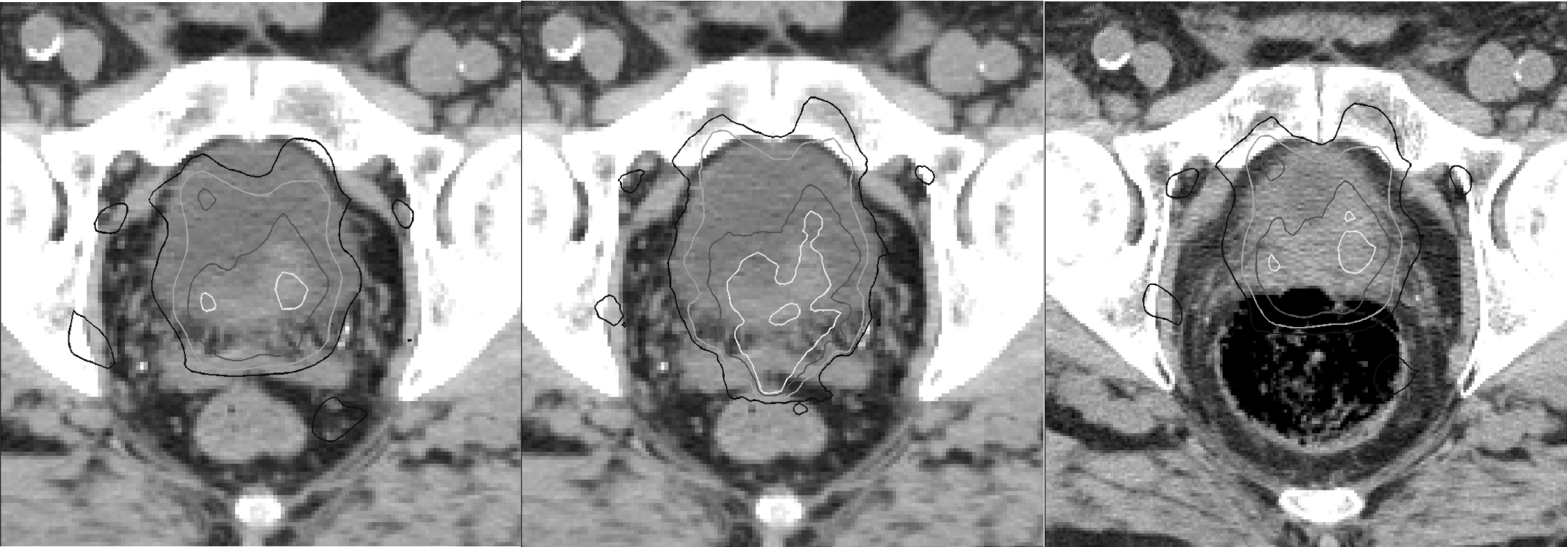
An example of dose deformation for a fraction with a large organ deformation, showing the planned (left) and deformed (middle) doses overlaid on the plan CT and the reconstructed dose (right) overlaid on the daily CT. Isodose lines are 105% (white), 100% (dark gray), 90% (light gray), and 80% (black) of the prescription dose.
